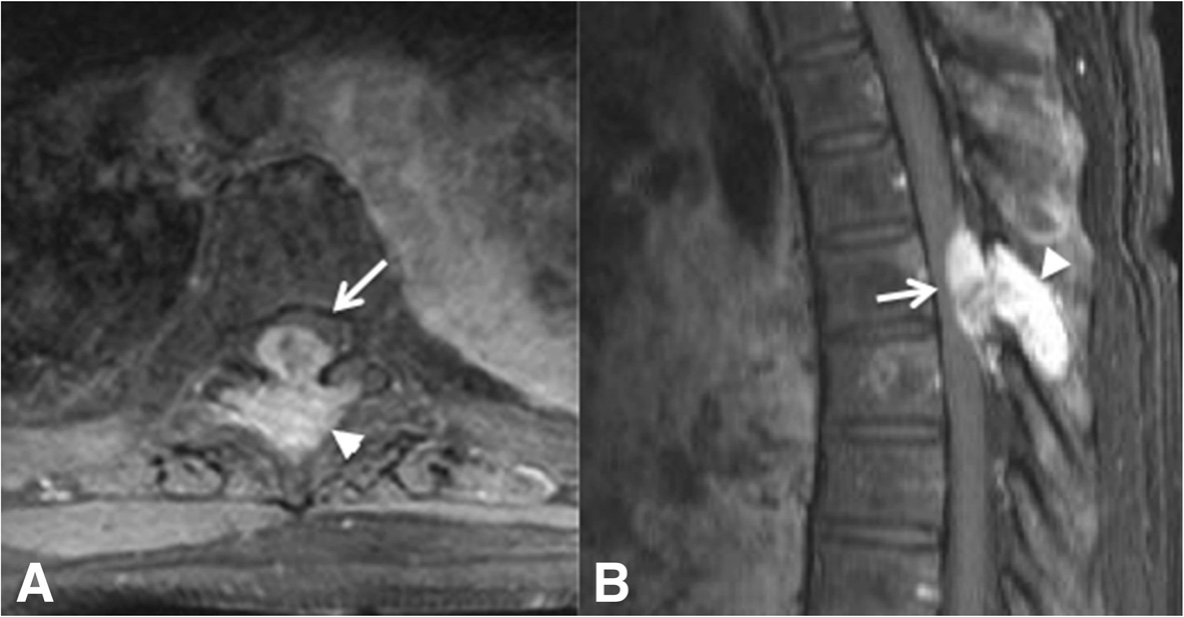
A 61-year-old woman with breast cancer metastatic to the spine leading to spinal cord compression syndrome. Axial (A) and sagittal (B) post-contrast T1-weighted MR images of a patient with metastatic breast cancer showing a bone lesion in the posterior elements of T6 (arrowhead), with high T2 signal intensity and intense contrast enhancement, impressing upon the spinal canal and dislocating the cord anterolaterally (arrow).
